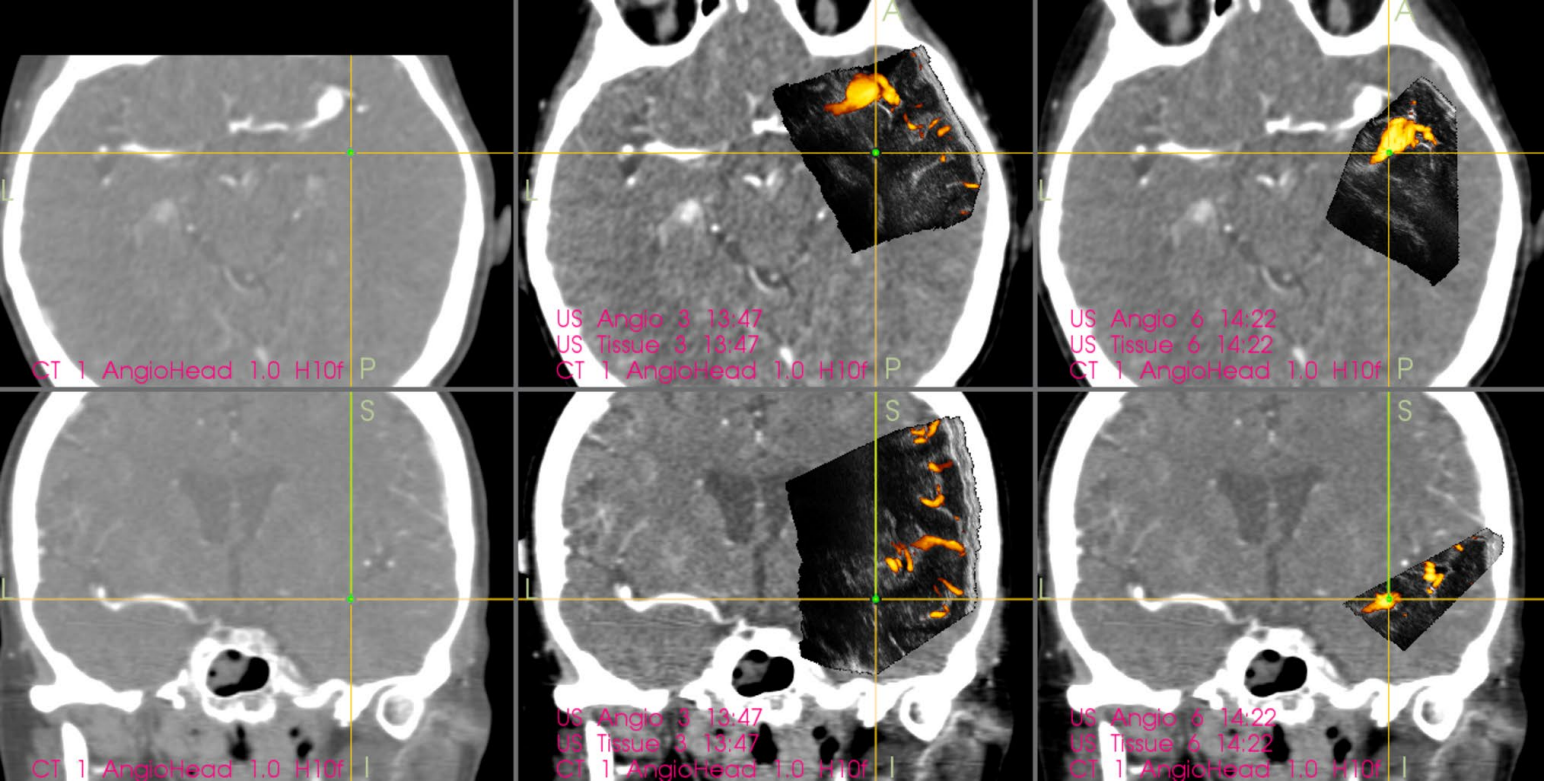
Example of an MCA aneurysm distinctly depicted by the navigated intraoperative 3D-US power-Doppler angiography. Left column: Preoperative navigation CT angiography showing an unruptured right-sided MCA aneurysm. (case No 18). Middle column: Initial 3D-US power-Doppler image acquired before the start of dissection; note the aneurysm is almost in the same location as depicted on preoperative CT angiography. Right column: Another 3D-US power-Doppler image acquired after partial dissection of the Sylvian fissure, note the significant shift of the aneurysm. Center of the yellow cross is located in the area of the aneurysm neck
